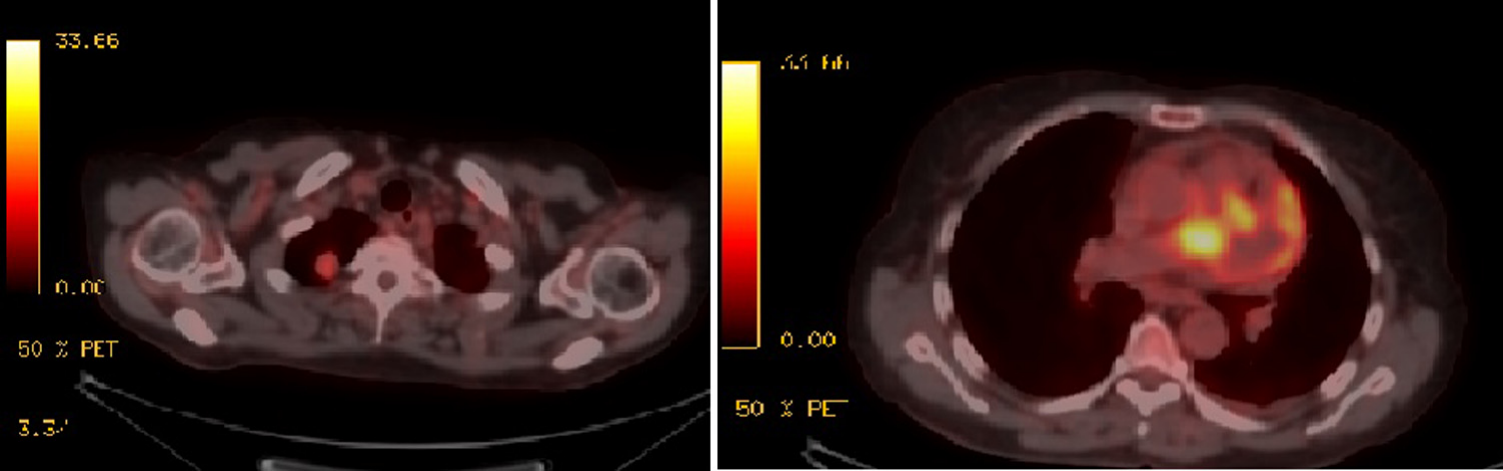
PET‐CT revealed an increased 18‐FDG‐standardized uptake value (SUV) of 4.0 in the right upper lobe nodule (left), associated with increased uptake of a soft tissue density mass in the perivascular space of the anterior mediastinum with a max SUV 10.9 (right).
